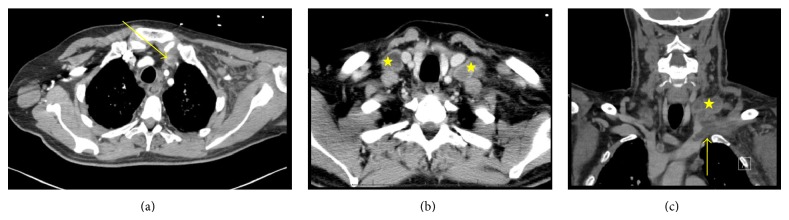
CT angiography showing (a) thrombus (arrow) in the left subclavian vein extending into the proximal innominate. Bilateral necrotic supraclavicular lymphadenopathy (star) does not show compression of the subclavian vein in the (b) axial and (c) coronal views.
